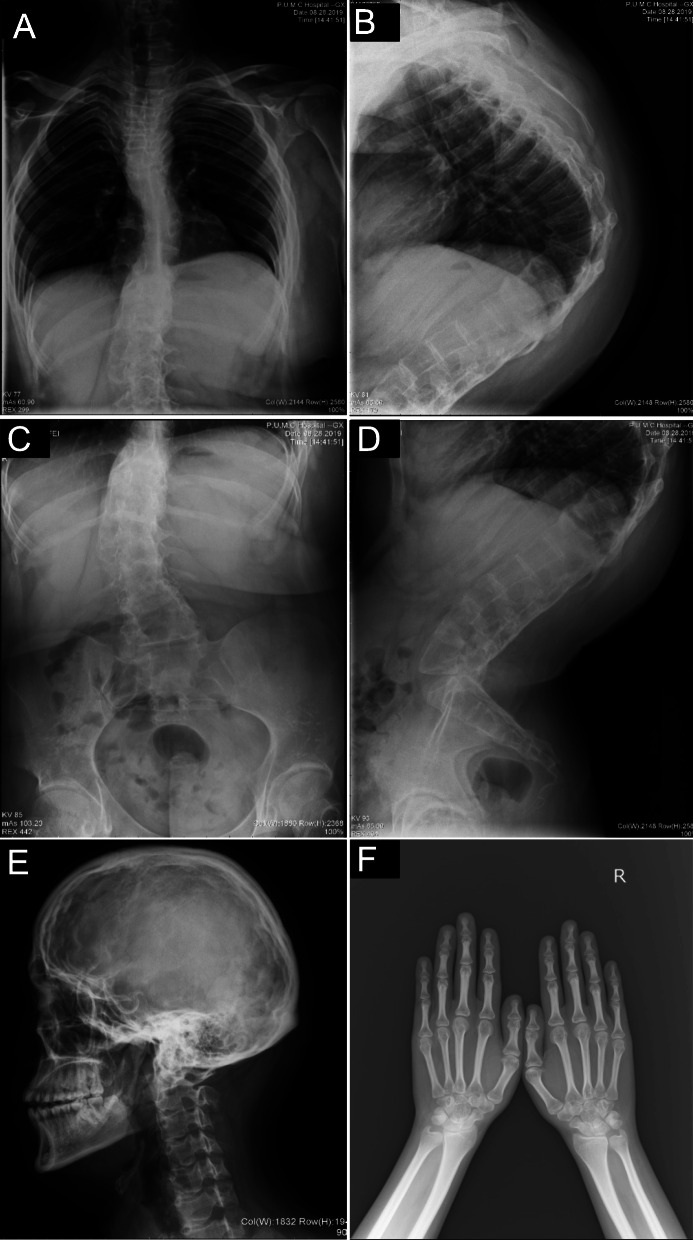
Radiographic findings of the patient at 17 years old. a X-ray of spine showing scoliosis and b kyphosis of thoracic vertebra, c scoliosis and d kyphosis of lumbar vertebra. e X-ray of skull showing copper-beaten appearance. f X-ray of hands showing osteopenia
